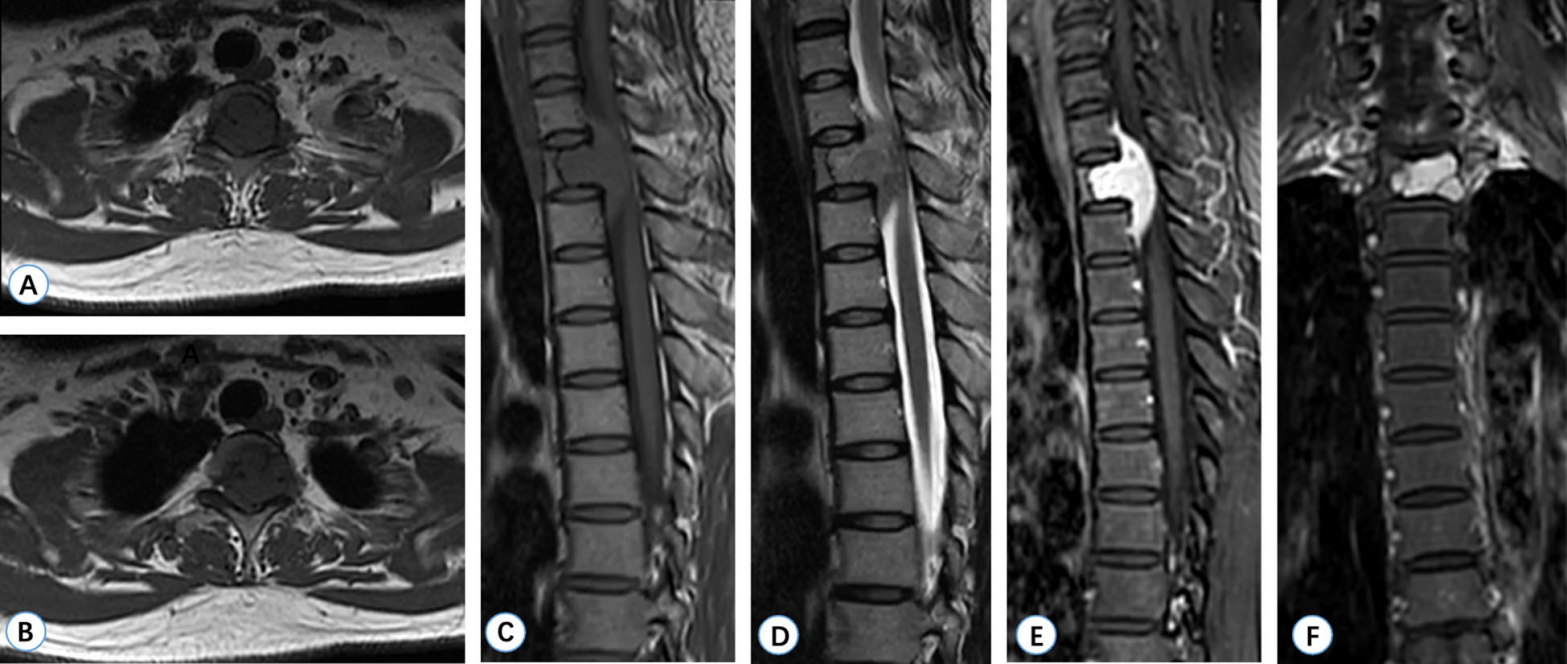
MRI manifestations of Case 1 (T2 vertebra). (A, B) Axial T1-weighted images reveal a lobulated, well-defined hypointense mass. (C) The sagittal T1-weighted image demonstrates that the tumor is isointense relative to the spinal cord. (D) The sagittal T2-weighted image indicates that the tumor exhibits high mixed intensity, accompanied by spinal cord compression. (E, F) Gadolinium-enhanced T1-weighted imaging displays significant irregular enhancement.
